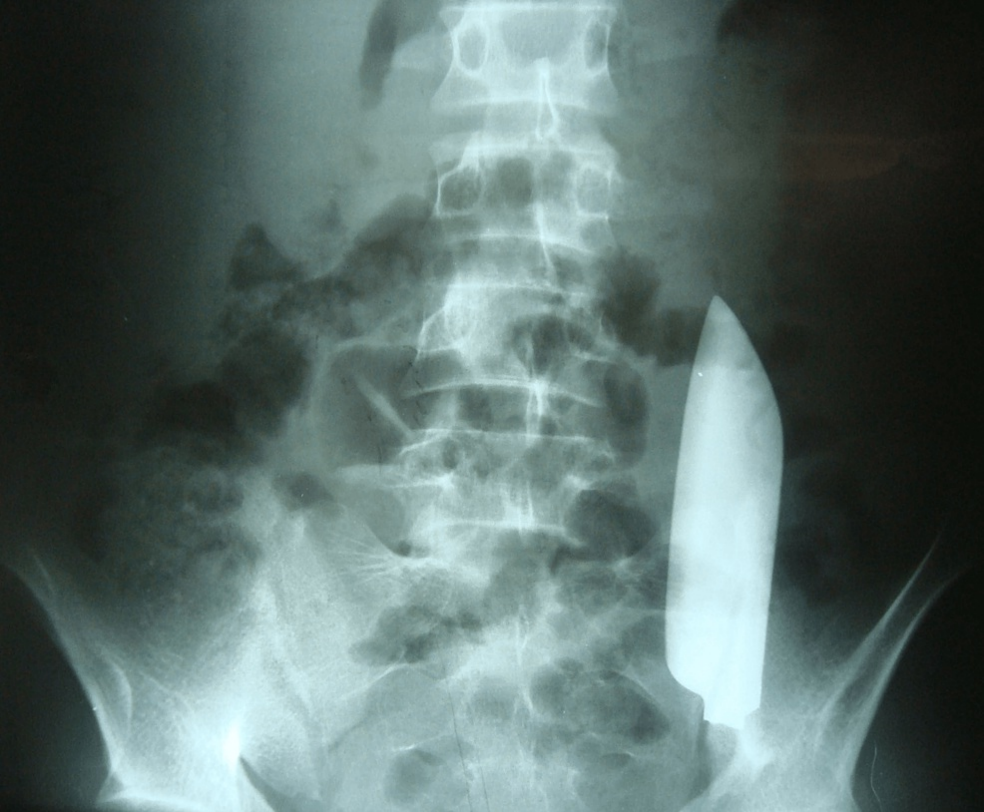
Abdominal X-ray in the posteroanterior view showing a free-floating metallic knife blade in the abdomen
